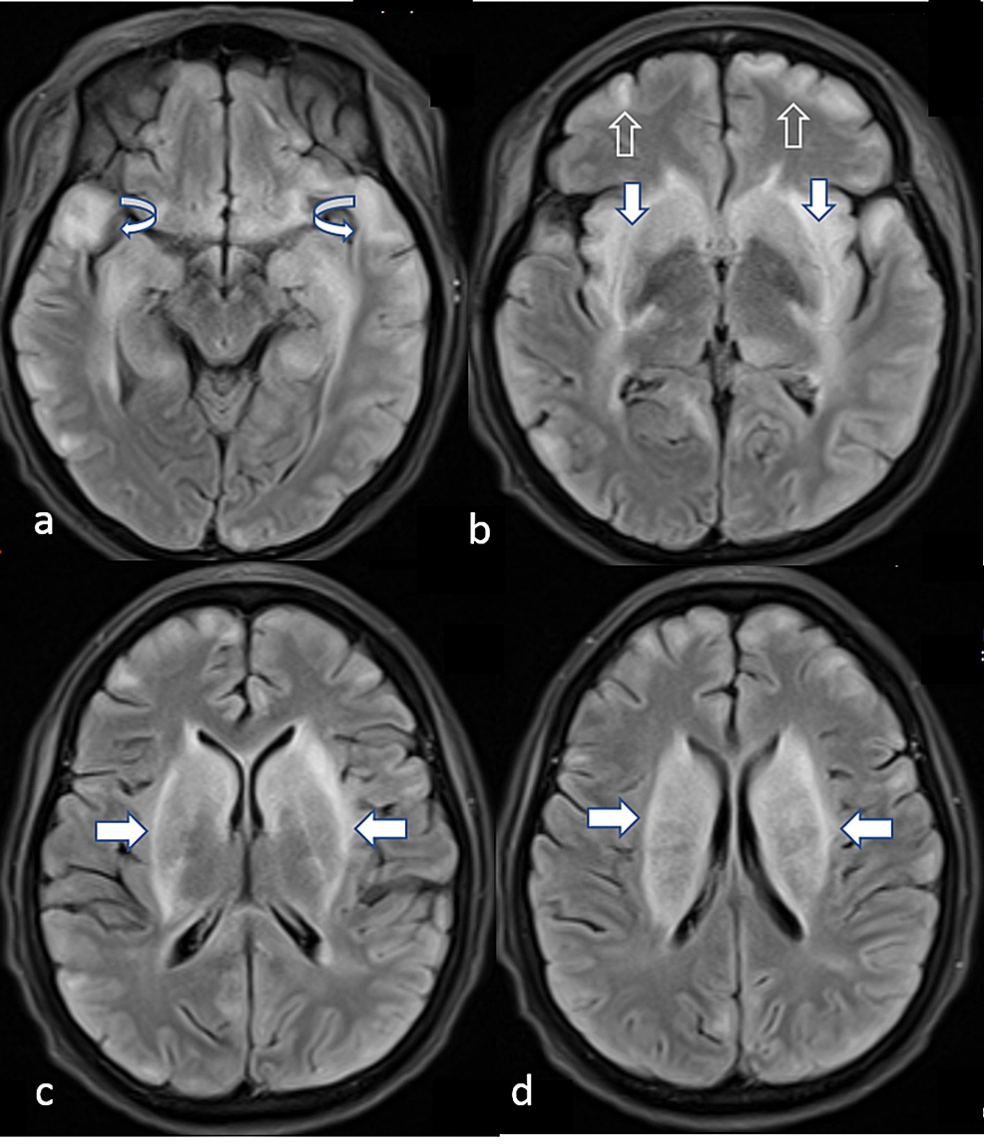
Pre-treatment MRI brain axial FLAIR. (a-d): Pre-treatment MRI brain axial FLAIR showing bilateral rather symmetrical basal ganglia external capsule (horizontal and vertical white arrows) and subcortical white matter (hollow and curved arrows) hyperintense areas in keeping with neuropsychiatric lupus with antibody-mediated striatal encephalitis. FLAIR, fluid-attenuated inversion recovery
